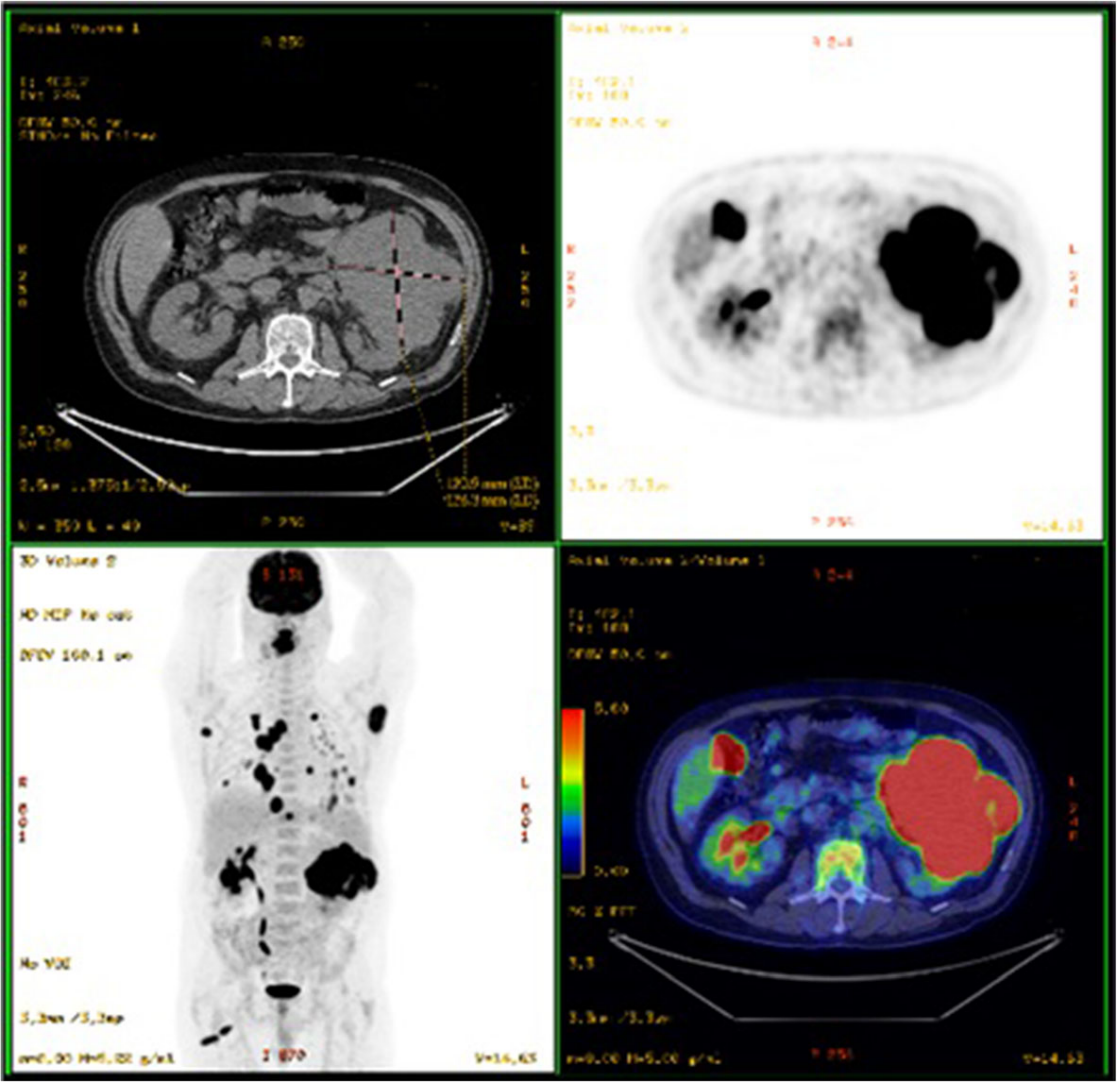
Positron emission tomographic-computed tomography scan shows a left kidney mass
